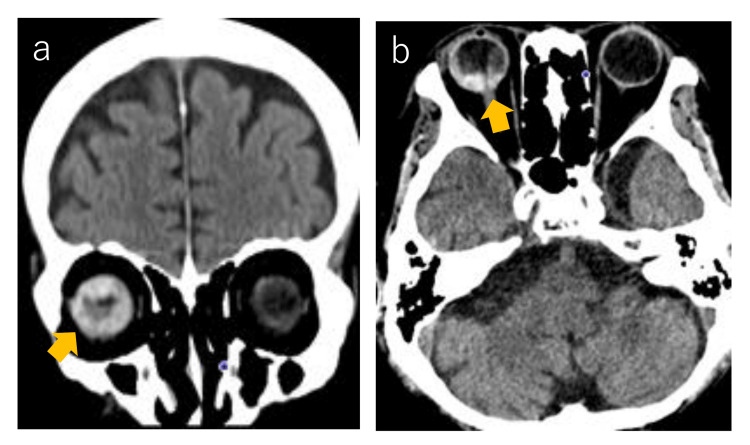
Brain CT findings Coronal (a) and axial (b) sections of the CT are shown. A highly absorbing mass was found in the right eyeball (yellow arrow). Whole-body CT images showed no apparent distant metastases other than the ocular tumor.
